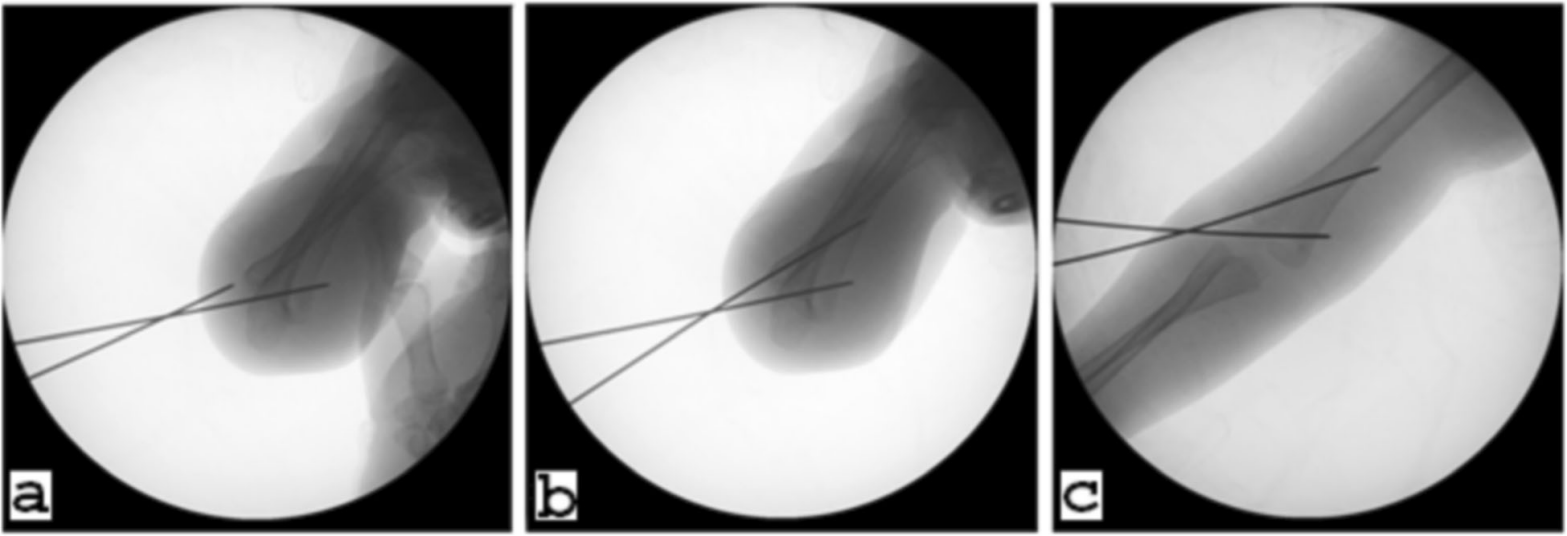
Placement of the proximal lateral pin was verified under Jones radiograph. a After satisfactorily placing the distal lateral pin, the proximal lateral pin was placed from the lateral distal cortex and aimed to exit in zone + 1 in the medial proximal cortex. b Trajectory of the proximal lateral pin within the distal humerus. c The proximal lateral pin exits the proximal medial cortex of the humerus
